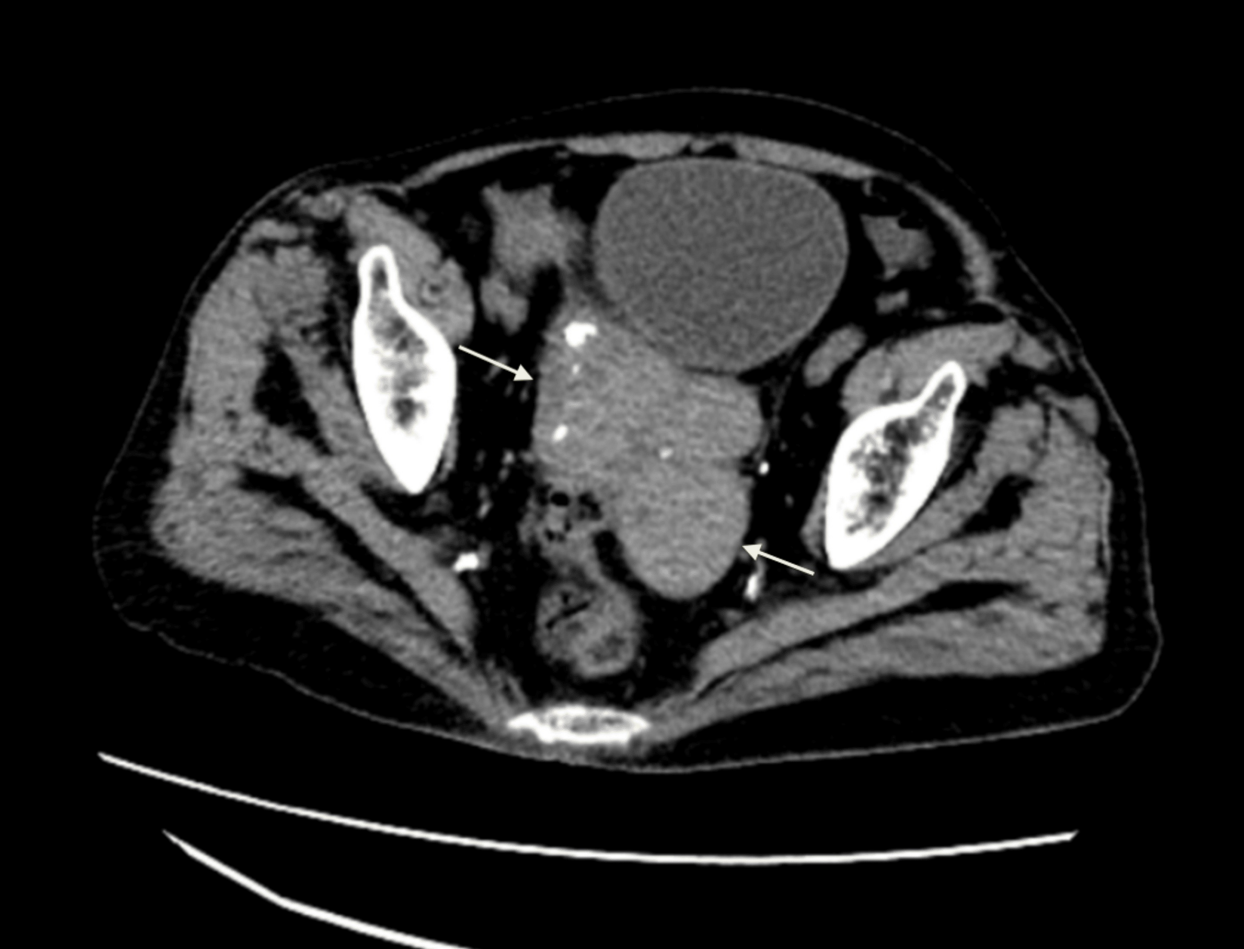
Axial view of the CT scan of the abdomen and pelvis shows a well-lobulated enhancing lesion of size 14.8 cm x 5 cm x 6.5 cm with a few small subcentimeter-sized foci of calcification noted arising from the terminal ileum bowel loops.
